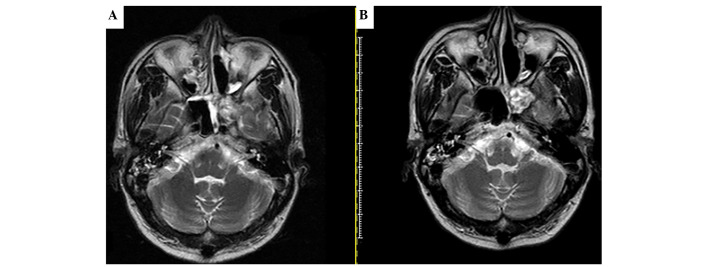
(A) MRI scan following two cycles of comprehensive chemotherapy and immunotherapy treatment. (B) MRI scan following four cycles of comprehensive chemotherapy and immunotherapy treatment, which determined that the patient had achieved a stable disease state, according to the Response Evaluation Criteria in Solid Tumors guidelines. MRI, magnetic resonance imaging.
